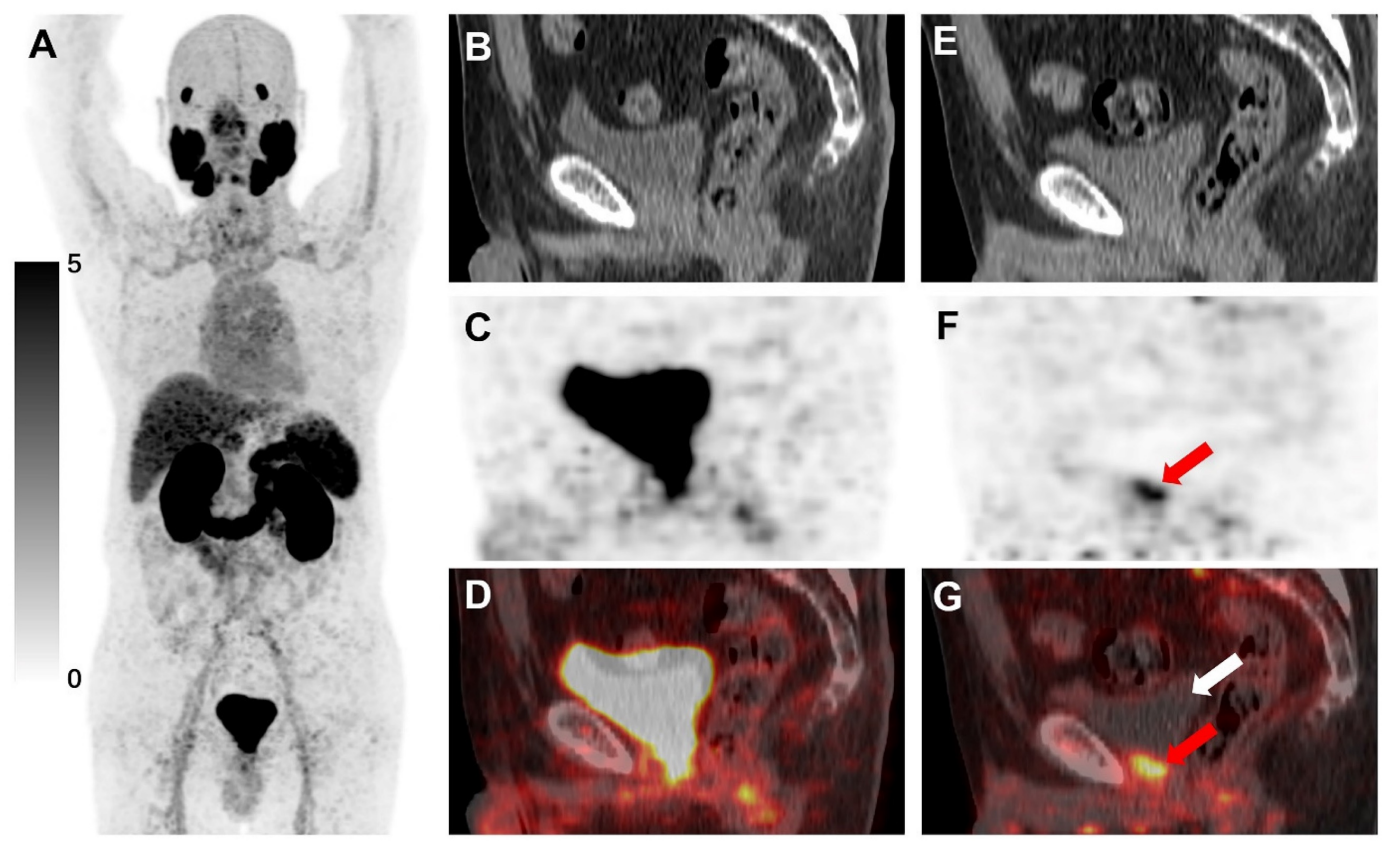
[^18^F]AlF-Thretide PET/CT images were acquired from a 64-year-old PCa patient with biochemical recurrence after radical prostatectomy. His total PSA level was 4.16 ng/mL one day prior to the PET/CT scan. The images showed focal tracer accumulation at the vesicourethral anastomosis site. (A-D) This accumulation closely resembled the urinary uptake in the routine PET/CT scan conducted 73 min post-injection, as shown in both the MIP image and the sagittal PET/CT images. (E-G) The early PET/CT scan, conducted 180 s post-injection, revealed a lesion in the same area with significant tracer uptake (red arrow, SUVmax 5.5). Notably, no tracer uptake was observed in the urinary bladder at this time (white arrow). A subsequent biopsy confirmed that this lesion was a local recurrence of PCa.
